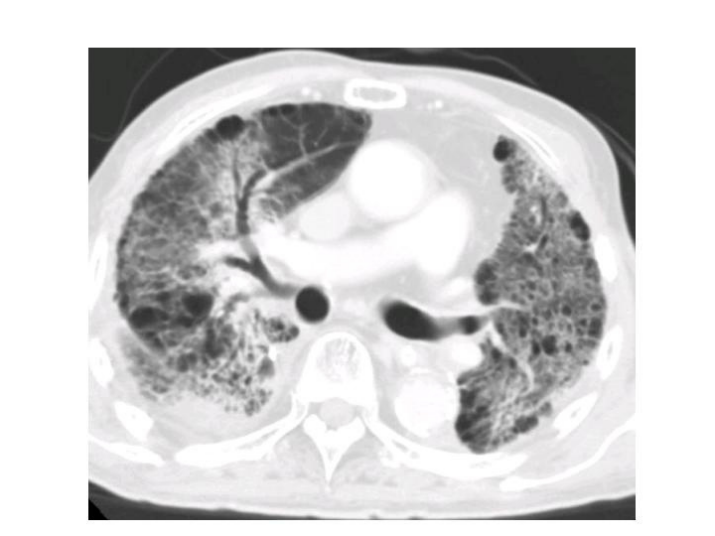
CT image of RP (patient No. 13).
